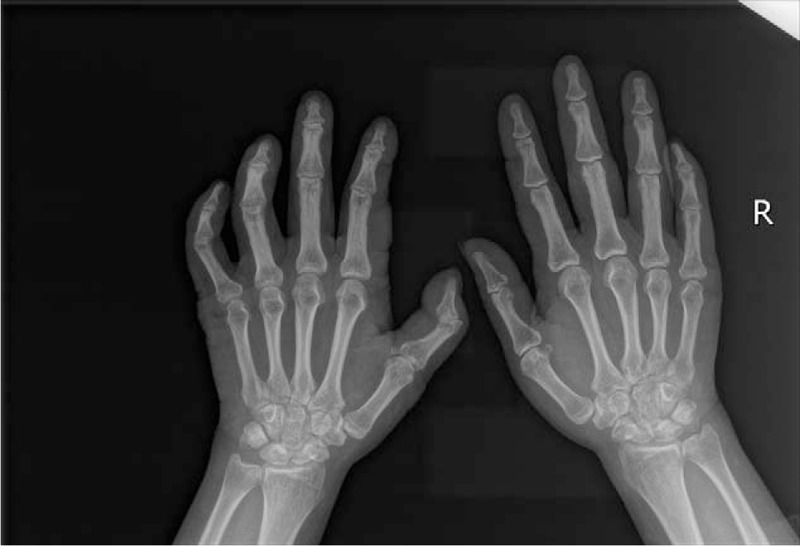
X-ray of the hands: no bone destruction, the bone density of the metacarpophalangeal joints was reduced, the joint space was normal.
